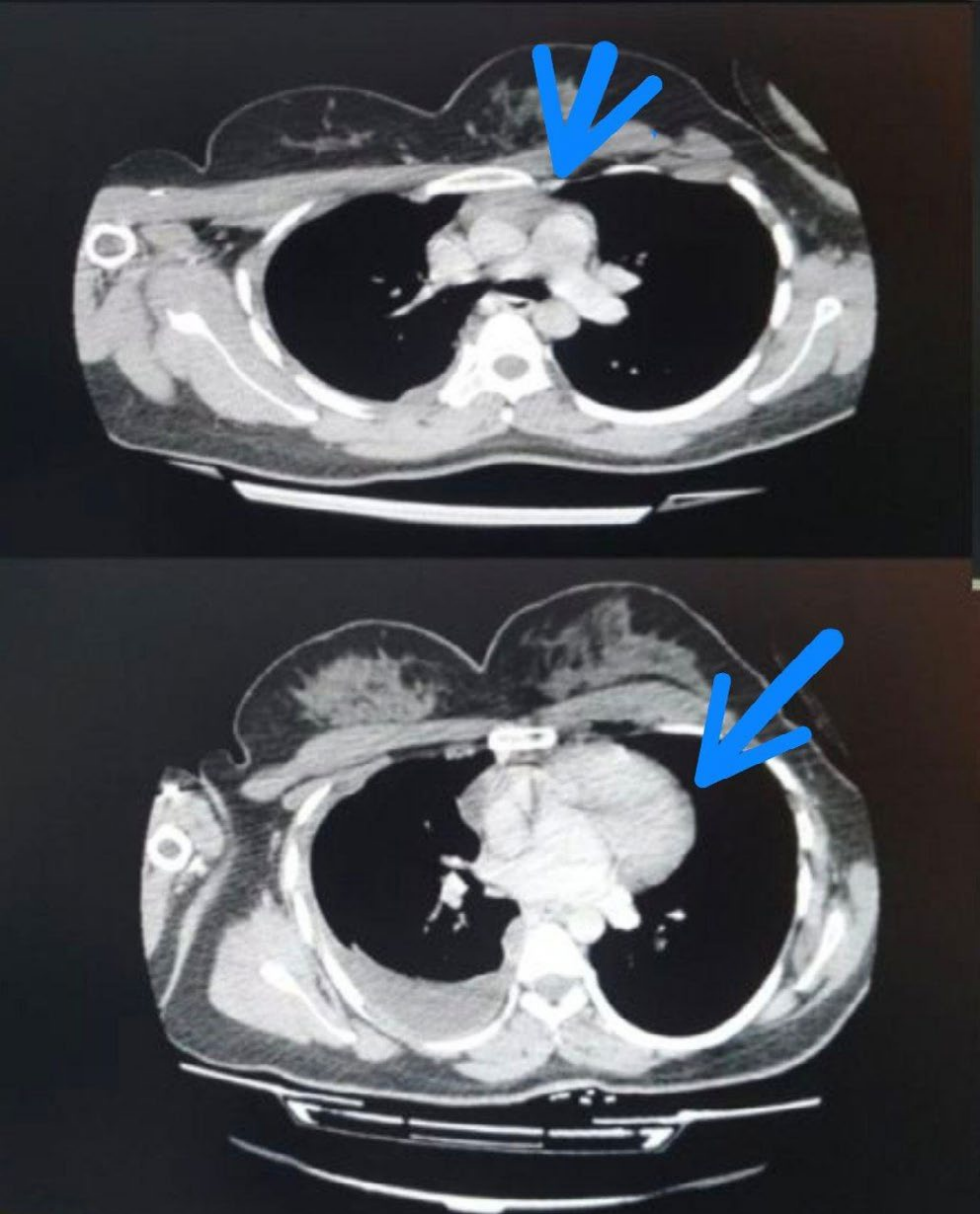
Contrast‐enhanced axial chest CT images (mediastinal window) show a well‐circumscribed, homogeneous, low‐attenuation pleural‐based lesion abutting the mediastinum, consistent with a cystic lesion. Additionally, a moderate associated pleural effusion is noted.
